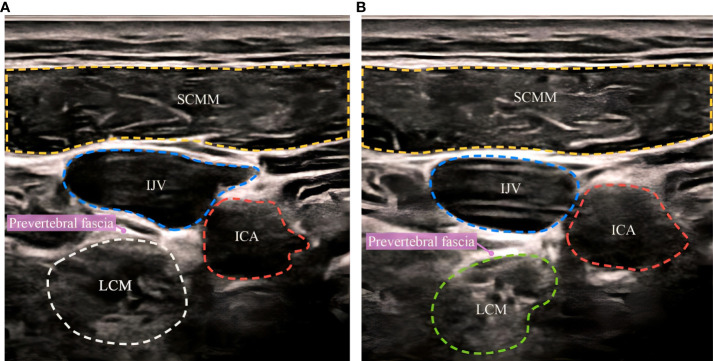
Ultrasound-guided stellate ganglion block before and after. (A) before SGB, (B) after SGB. SCMM, sternocleidomastoid muscle; IJV, internal jugular vein; ICA, internal carotid artery; LCM, longus collimuscle.
